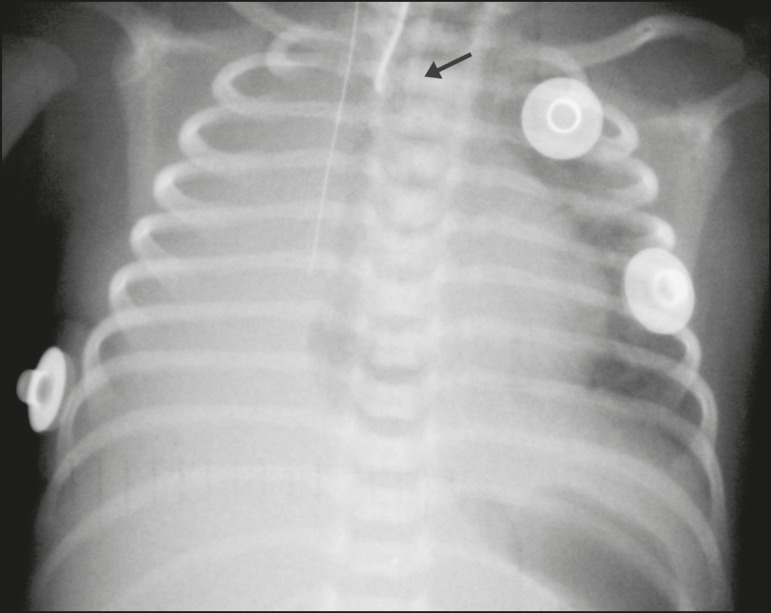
Chest X-ray of a neonate born at 33 weeks of gestation with a birth
